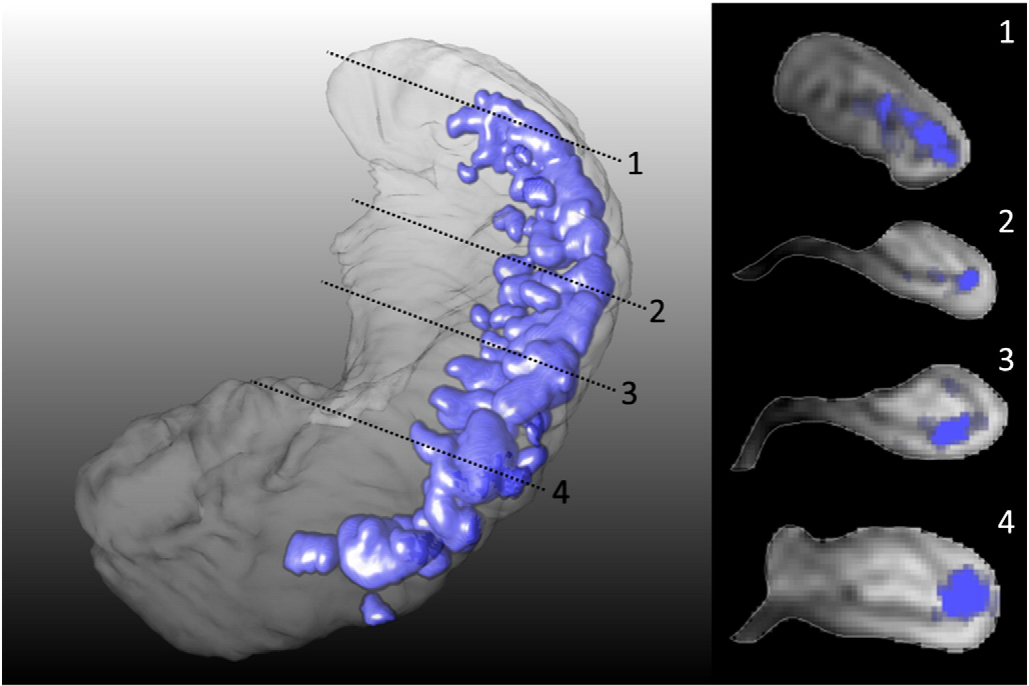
3D representation of hippocampal T2 hyperintensities. Left: probabilistic segmentations of FLAIR hypointense HT2Hs of a representational subset were registered to the hippocampus of one subject. The 3D distribution of HT2Hs is shown in purple, all FLAIR hypointense HT2Hs were located in the vestigial sulcus in the full length of the hippocampus, and a vast majority in the ventrolateral flexion points of CA1. Right: cross-sections of the hippocampus shown on the left.
